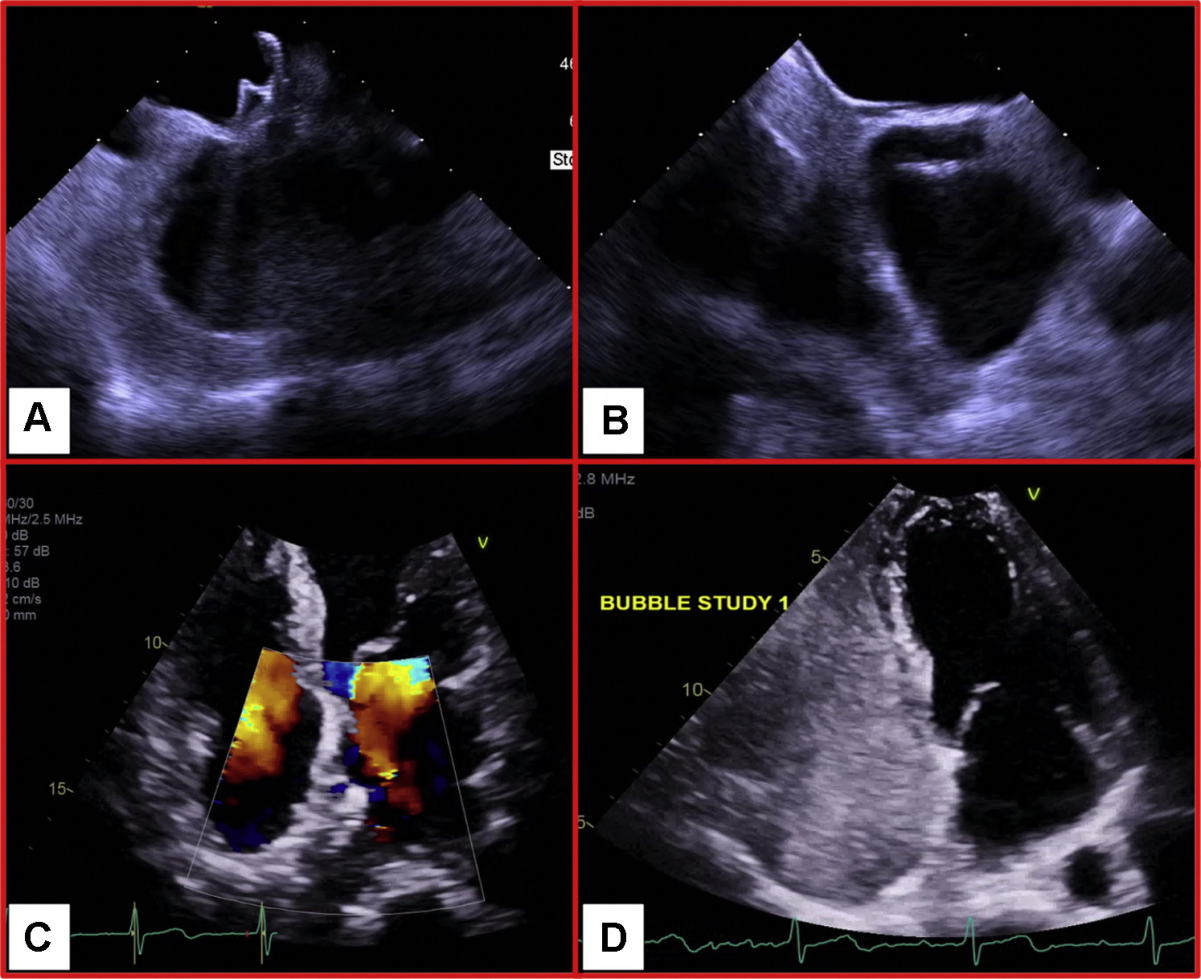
Post-Deployment Results (A, B) Post-deployment intracardiac echocardiography images showing atrial septal occluder to be adequately sandwiched in the atrial septum. (C) Transesophageal echocardiography on postoperative day 1 showing trivial interatrial doppler flow through the septum. (D) Bubble study with agitated saline showing no intracardiac shunt.
